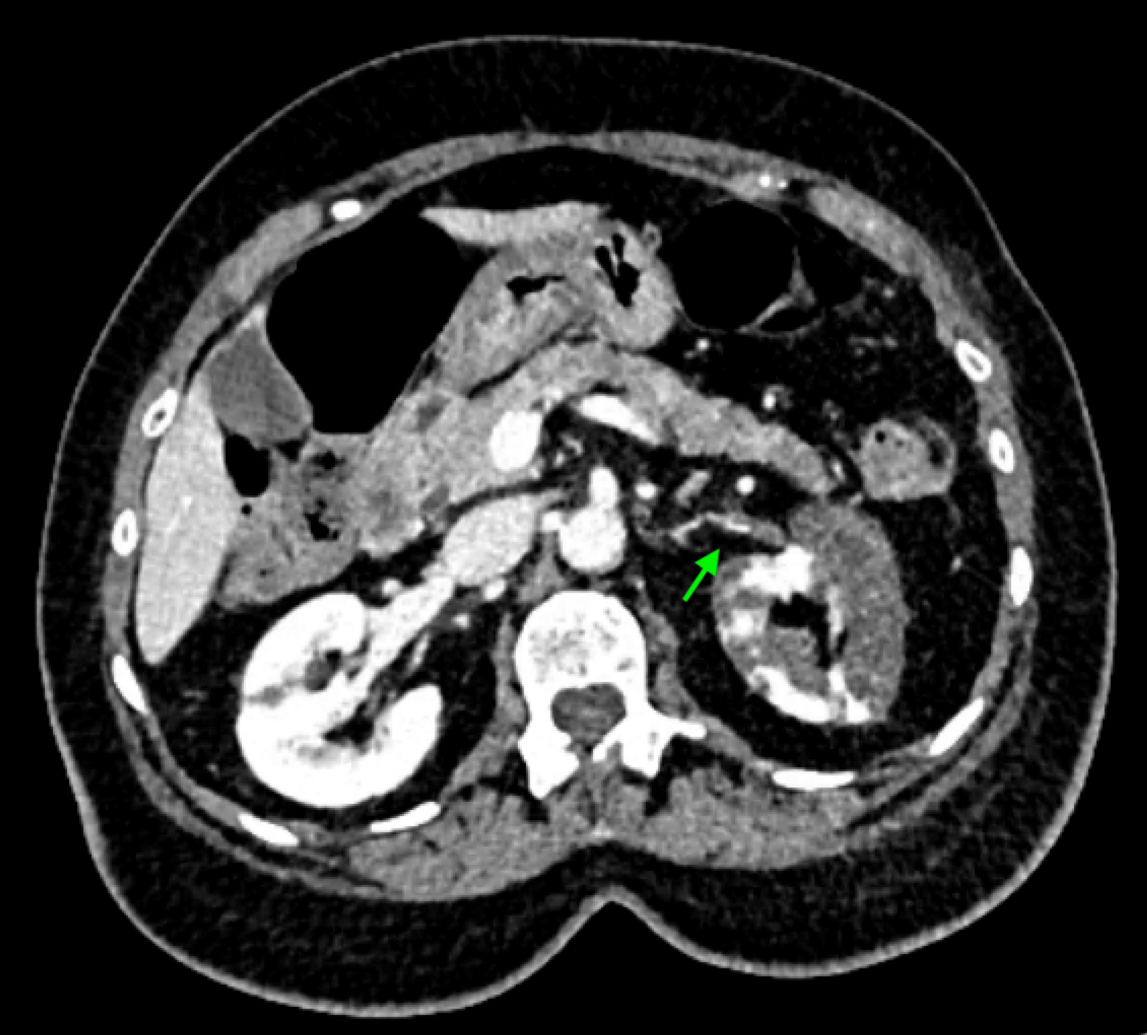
Axial contrast-enhanced CT abdomen and pelvis (CTAP) The green arrow highlights a filling defect in the left renal artery, consistent with left renal artery thrombosis. There is reduced enhancement of the left kidney compared to the right, suggesting impaired perfusion.
